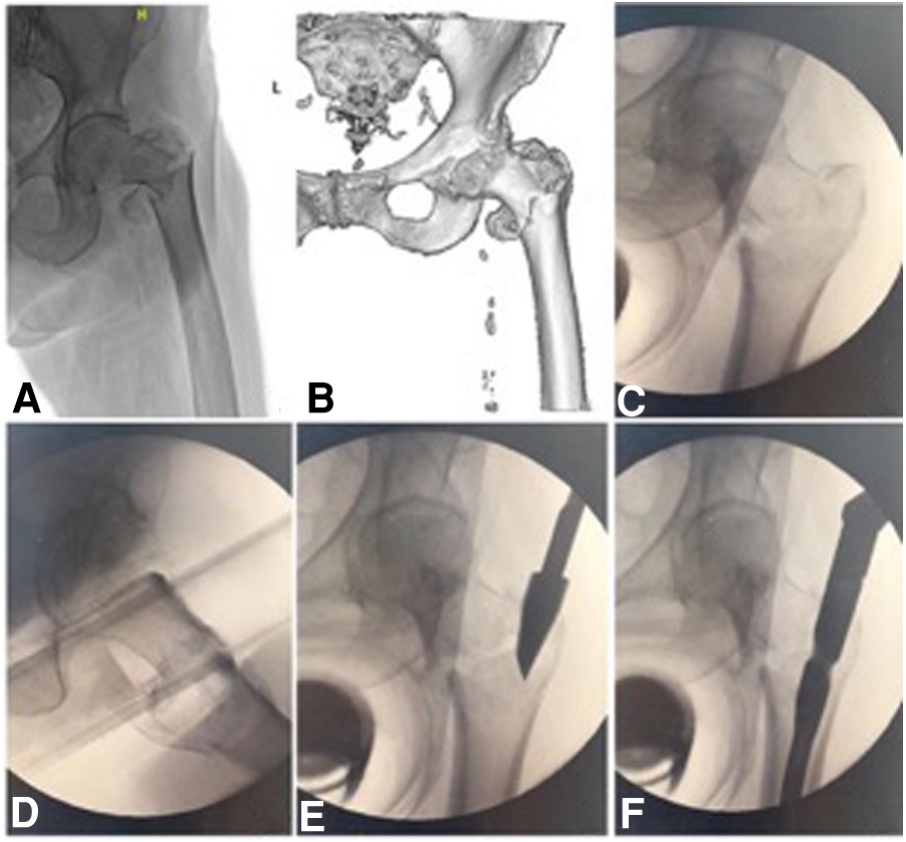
An 81-year-old woman with a comminuted intertrochanteric fracture, involving the medial arch and lateral wall was graded as AO/OTA 31-A2.2 (2018 edition). Preoperative x-ray (A) and CT scan (B) show the comminuted intertrochanteric fracture. (C,D), anteroposterior and lateral images show that the reduction was acceptable under axis traction. (E,F) The entry point was confirmed, and the displaced medial arch fragments developed after the femoral nail insertion.
